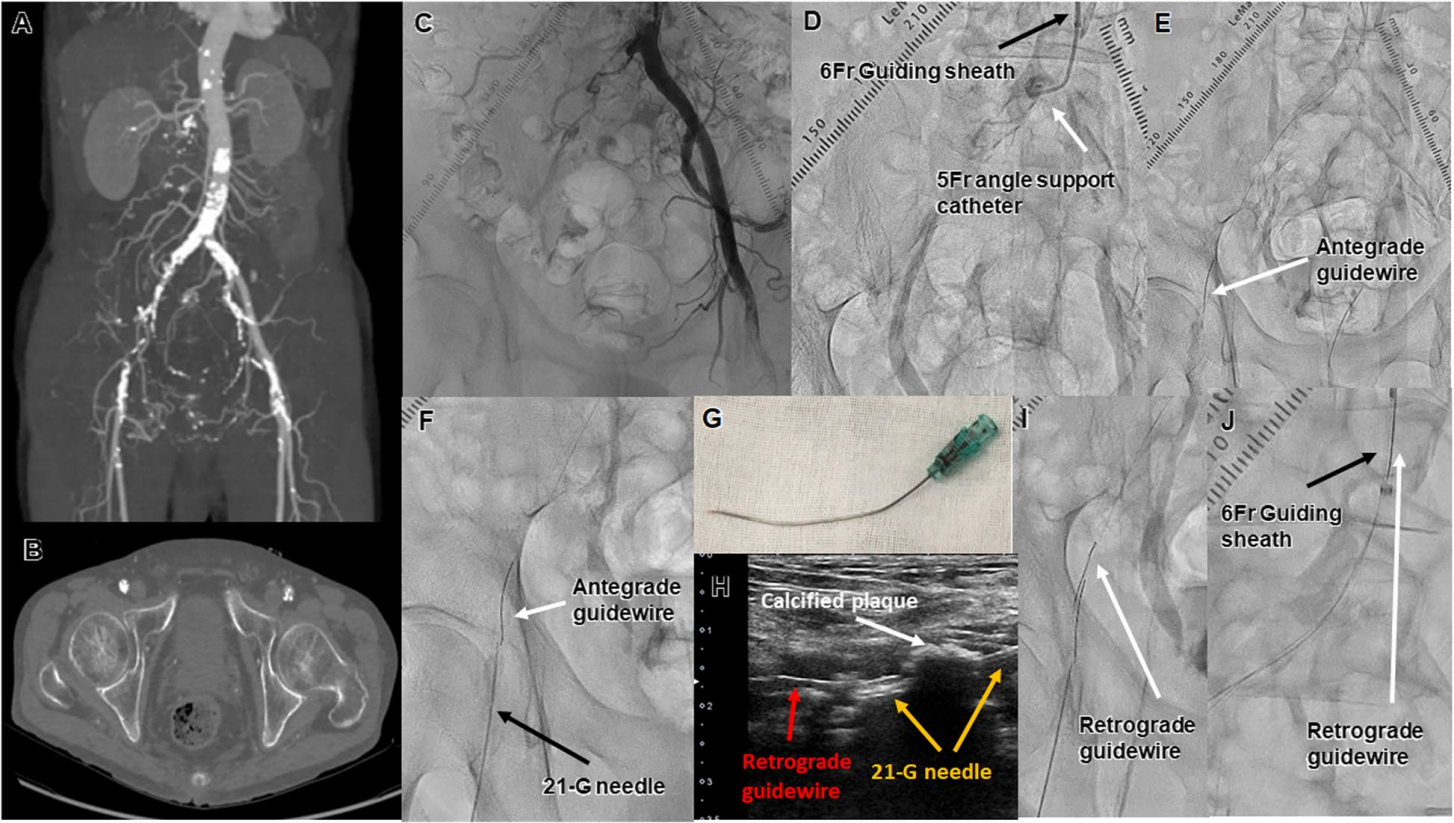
Case 2. A, B Preprocedural enhanced computed tomography images. C Control angiography showed total occlusion from the proximal aspect of the common iliac artery. D The black arrow shows the 6Fr guiding sheath. The red arrow shows the 5Fr angled support catheter. E The antegrade guidewire was advanced into the distal external iliac artery. The white arrow shows the antegrade wire. F The white arrow shows the antegrade wire. The black arrow shows the 21-G needle. G Image of a 21-G needle created with a double bend. H Duplex ultrasound showed that the retrograde guidewire was inside the vessel. The yellow arrow shows the 21-G needle. The white arrow shows calcified plaque. The red arrow shows the retrograde guidewire. I Bidirectional wiring. The white arrow shows the retrograde guidewire. J The retrograde guidewire was advanced into the antegrade guiding sheath to achieve guidewire externalization. The black arrow shows the antegrade 6Fr guiding sheath. The white arrow shows the retrograde guidewire
